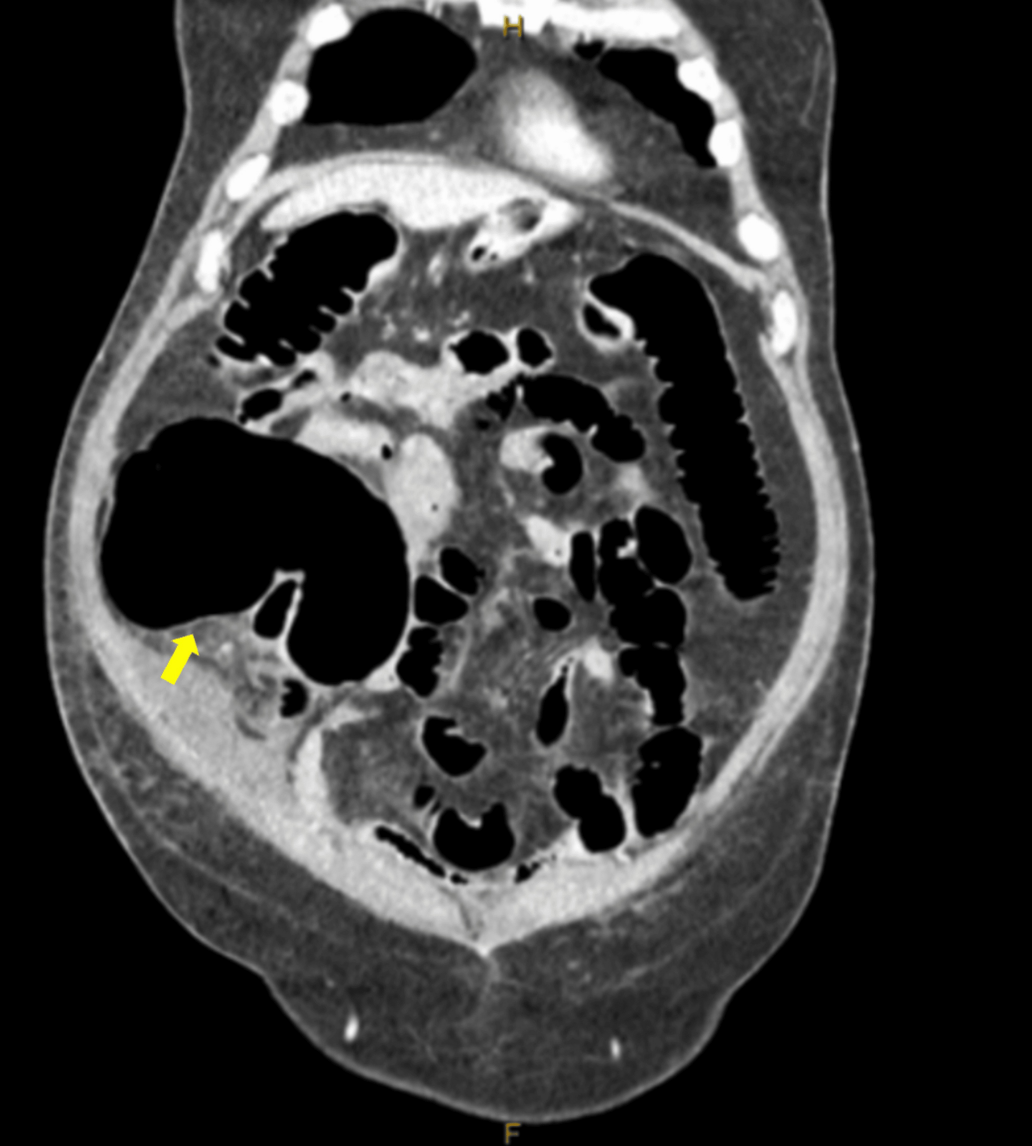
Coronal CT abdomen pelvis demonstrating a distended right colon, especially the cecum (yellow arrow)
